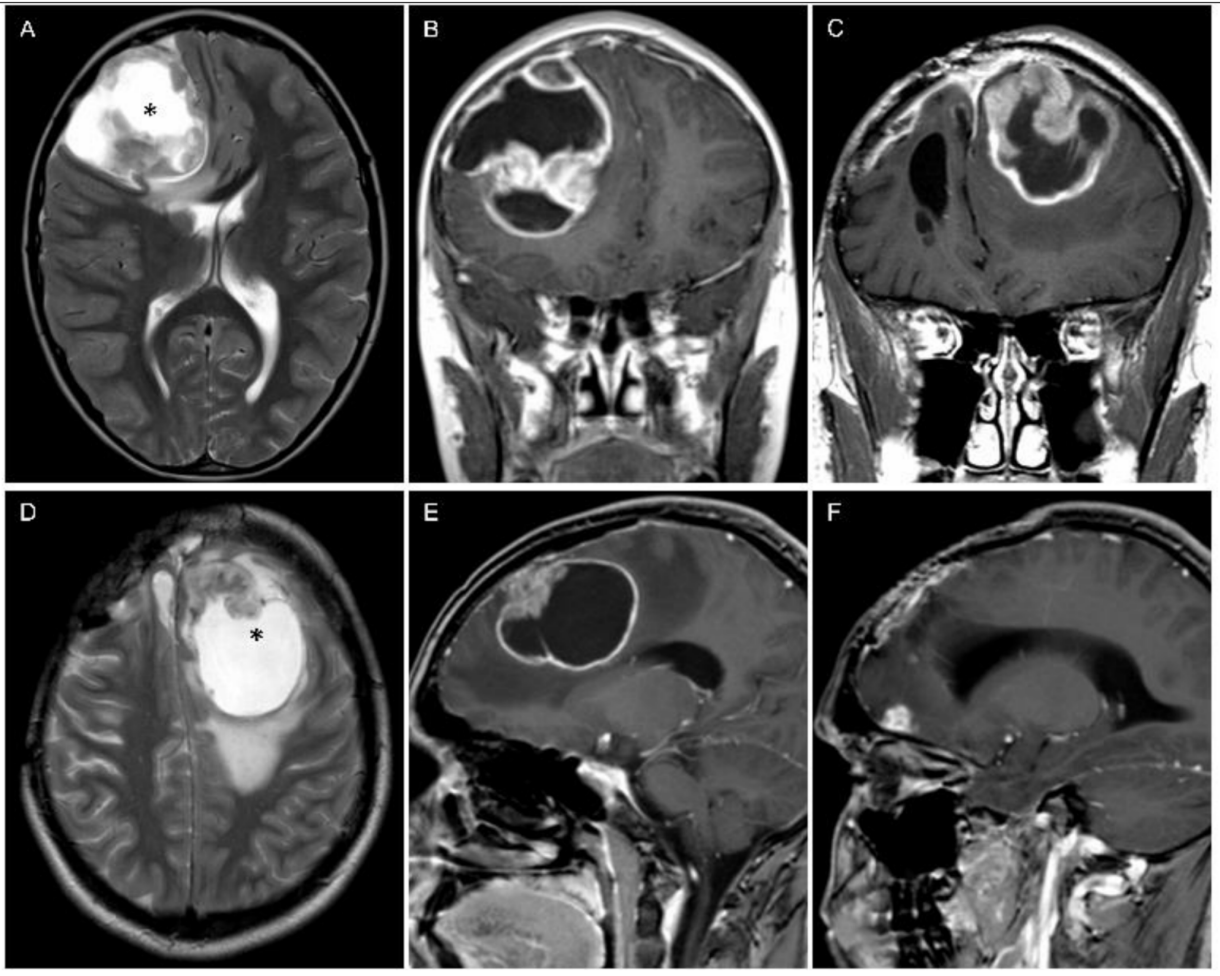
A 9-year-old boy with a *ZFTA*-fusion positive supratentorial ependymoma in the right frontal lobe at diagnosis on T2-weighted (A) and post-contrast T1-weighted images (B). The patient had at first relapse a local recurrent tumor 43 months after initial total resection. On follow-up two further local recurrences occurred. The local recurrent tumors never crossed the midline. (C-E) The fourth recurrence was a left sided leptomeningeal metastasis with very similar signal intensities on T2-weighted (D) and post-contrast T1-weighted images (C, E). The tumor tissue contained a large central non-solid area (*) at diagnosis (A) and in relapse (D). (E) shows the broad-based contact of the metastasis to the dura mater. (F) The patient simultaneously showed a second leptomeningeal metastasis at the right frontal base
